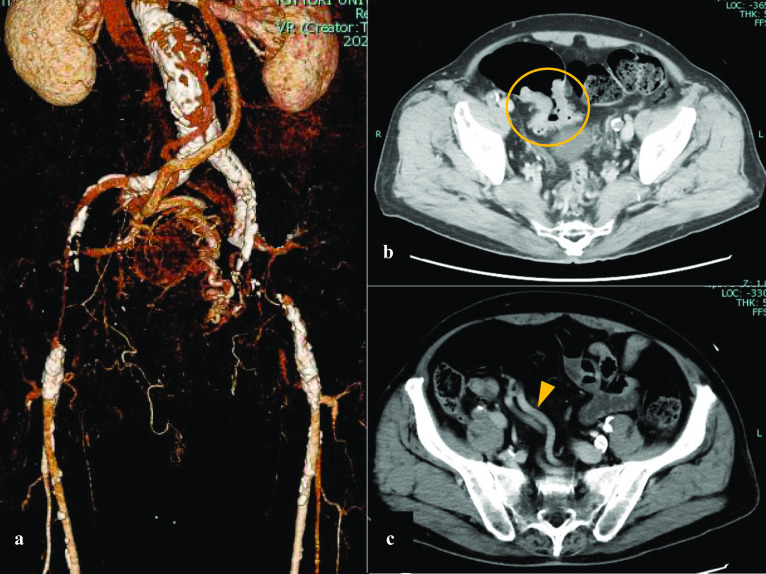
Computed tomography images from periodic surveillance for abdominal aortic aneurysm and asymptomatic peripheral artery disease. a Severe calcification was observed between the abdominal aorta and both femoral arteries. Both external iliac arteries were disrupted. b Well-demarcated wall thickening was found in the sigmoid colon. c The inferior mesenteric artery was well developed and measured more than 5 mm in diameter. A dilated inferior mesenteric vein was winding along the inferior mesenteric artery
